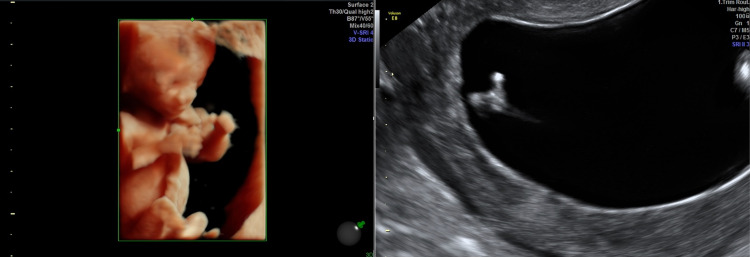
Three-dimensional (left) and two-dimensional (right) appearances of syndactyly on ultrasound.
